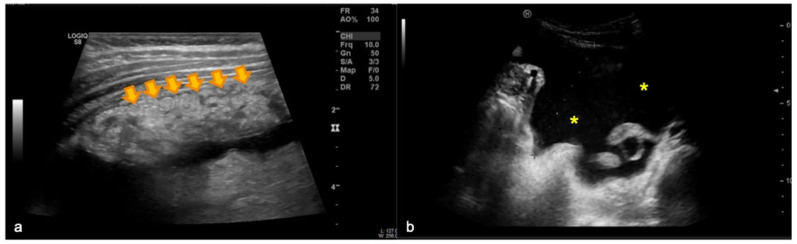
Abdominal ultrasound images of the 12-year-old patient showing the omental cake sign characterized by omental thickening and infiltration (orange arrows in a) and ascites (yellow asterisks in b).
